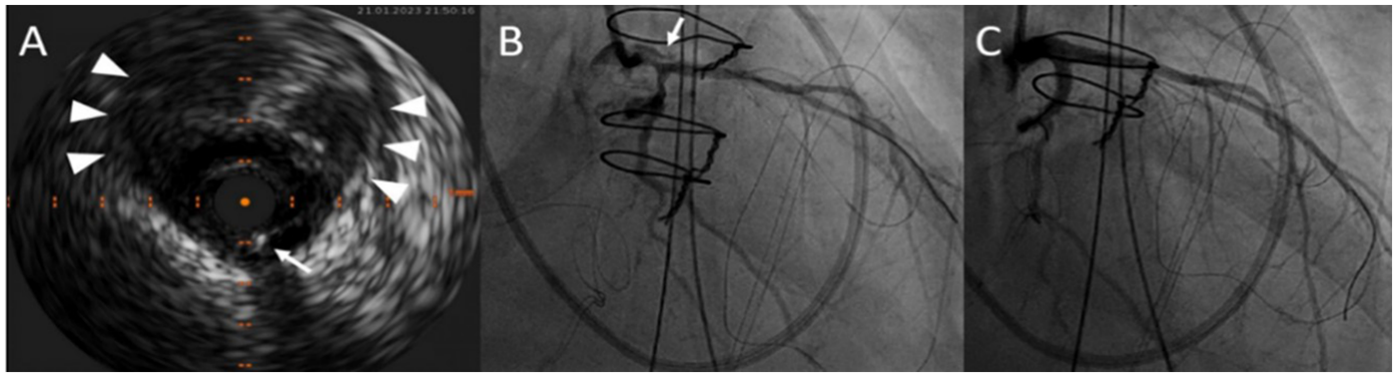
After wiring both the left anterior descending artery and the left circumflex artery, intravascular ultrasound of the left main coronary artery confirmed the correct wire position (white arrows) in the true lumen. The white arrowheads point at the large false lumen (A). RAO projection in the coronary angiogram displaying dissection narrowing of the left main ostium and white arrow points at the false lumen (B). Postoperative result after LAD and LCX stenting with a nano-crush technique (C).
